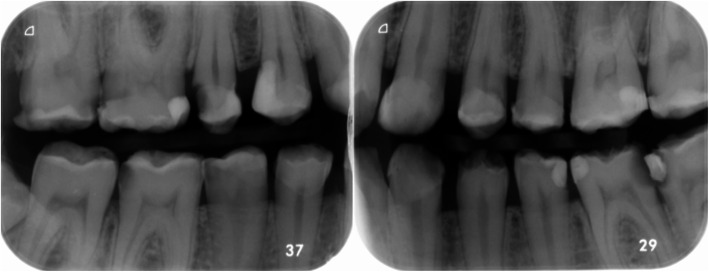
Bitewings right and left taken at 46 months followup
